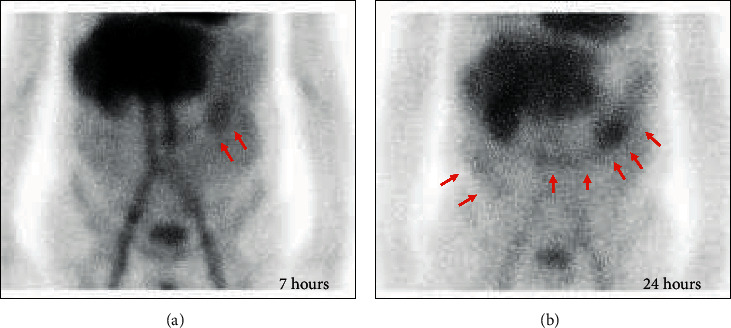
Scanning images of 99 mTc-labeled human serum albumin (HSA) scintigraphy. 99 mTc-labeled HSA scintigraphy revealed albumin leakage from the gastrointestinal tract (arrows). (a) At 7 hours of scanning, collection of the radiotracer was found in the left upper portion of the abdomen, indicating albumin loss into the small intestine. (b) At 24 hours of scanning, diffuse collection of the radiotracer was found in the right and upper portions of the abdomen, indicating albumin loss into the colon.
